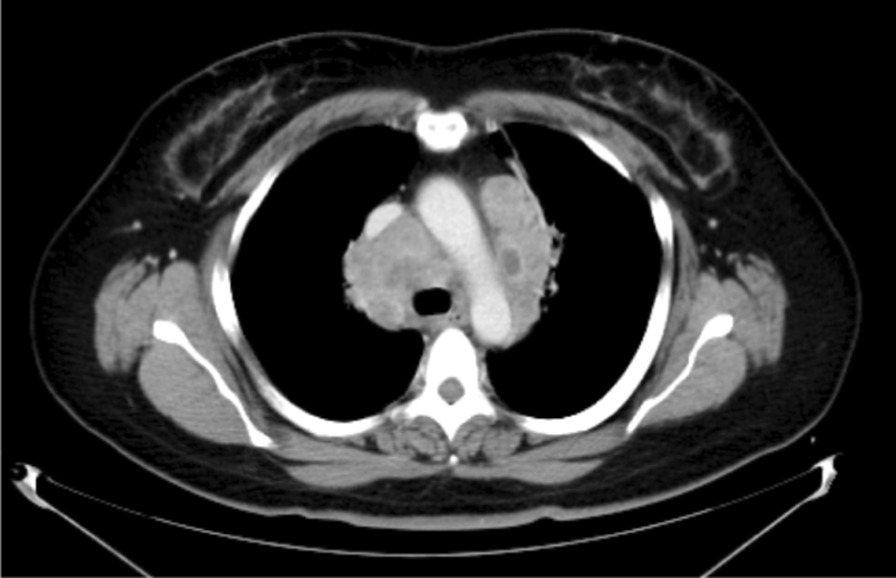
Thoracic computed tomography with contrast enhancement showing mediastinal lymph node swelling which contained low-density areas
